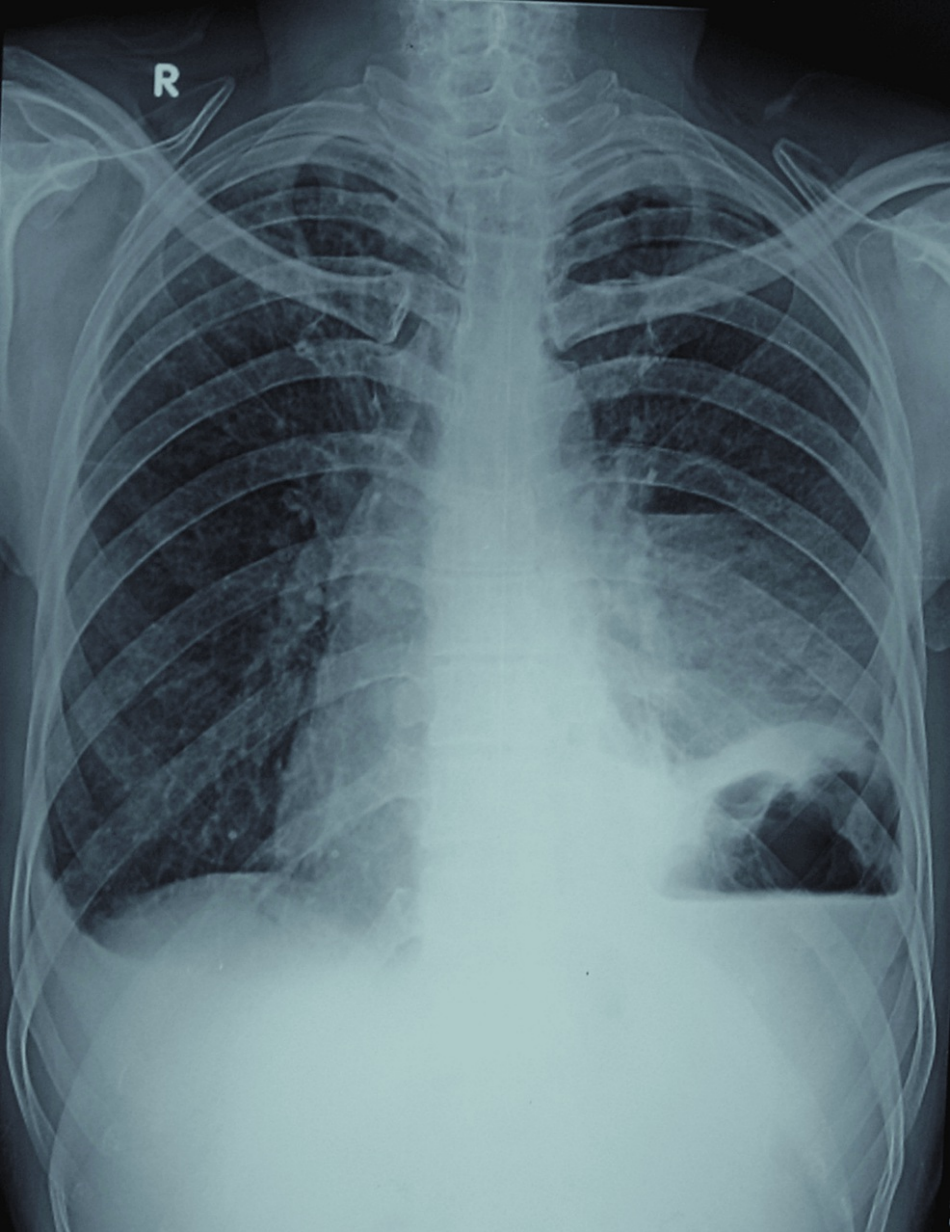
Chest X-ray showing reduced subcutaneous emphysema and pleural collection.
